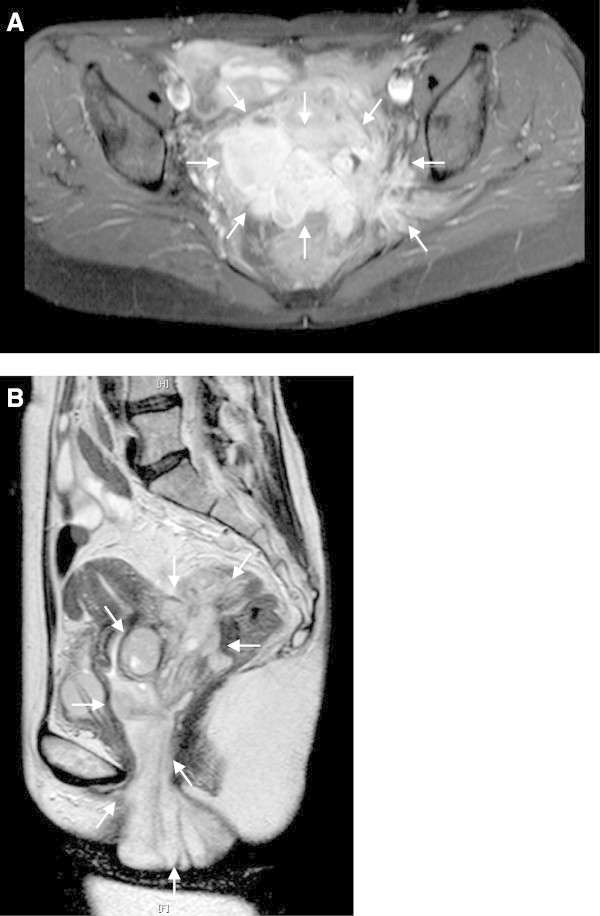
MRI images before the primary surgery. The tumor invaded the left pelvic wall (A; axial fat-saturated T1-weighted image) and the vagina (B; sagittal T2-weighted image).
